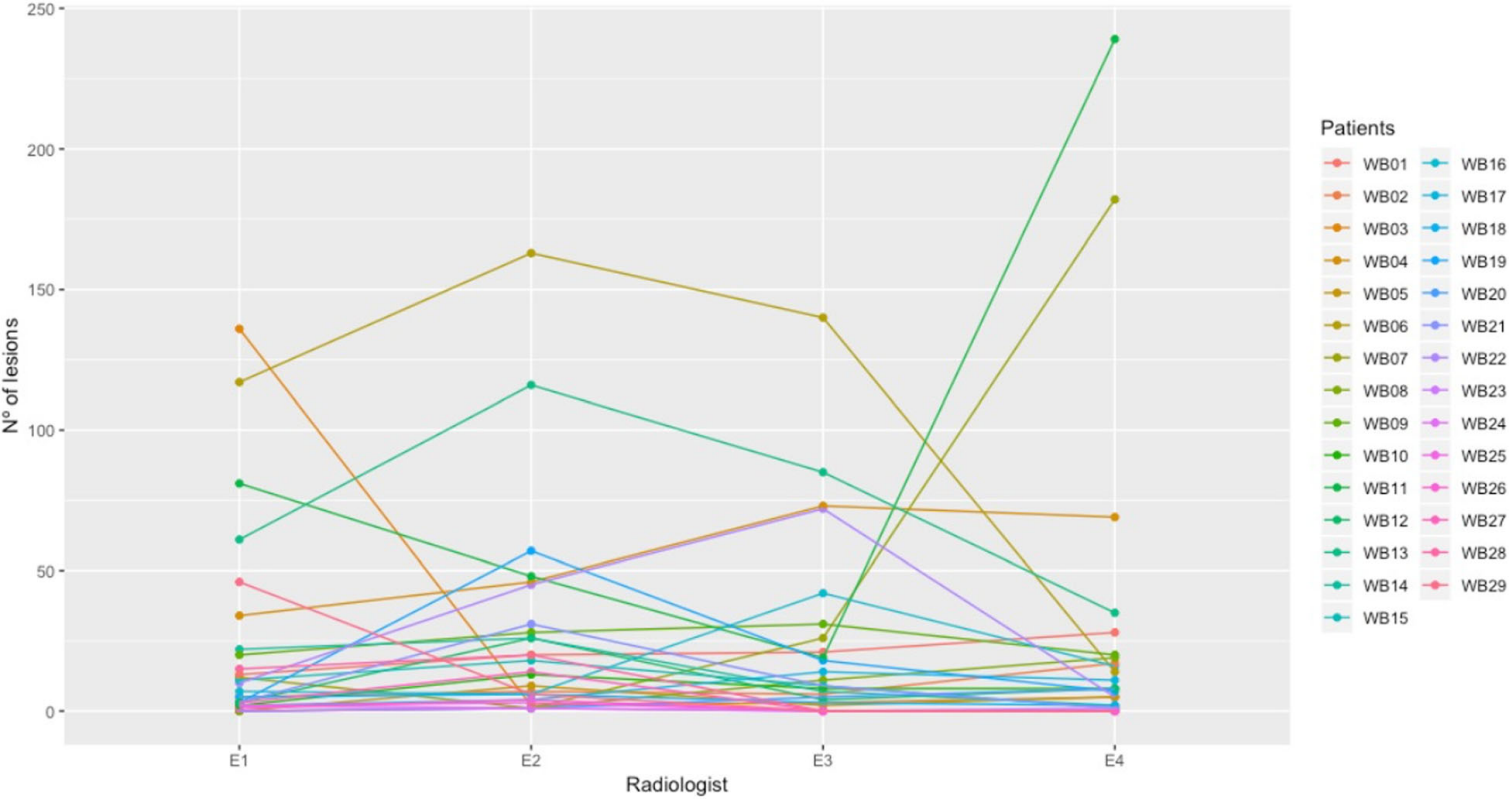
Parallel coordinate plot for the number of manually segmented lesions by each radiologist (E1, E2, E3, E4), per DWI (WB 01–22)
